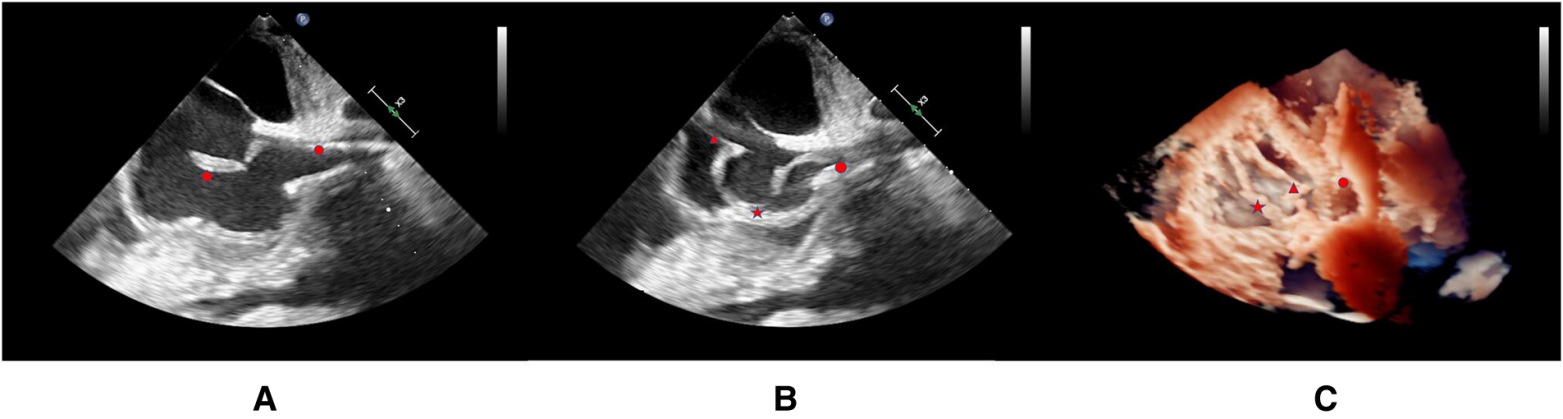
The locations of the floating thrombus, gooseneck catcher, and PICC are indicated in the image by the five-pointed star, triangle, and circle, respectively. (A) Location of thrombus under transesophageal ultrasound (B) Location of thrombus and snare under transesophageal ultrasound (C) 3D reconstruction after endovascular treatment.
